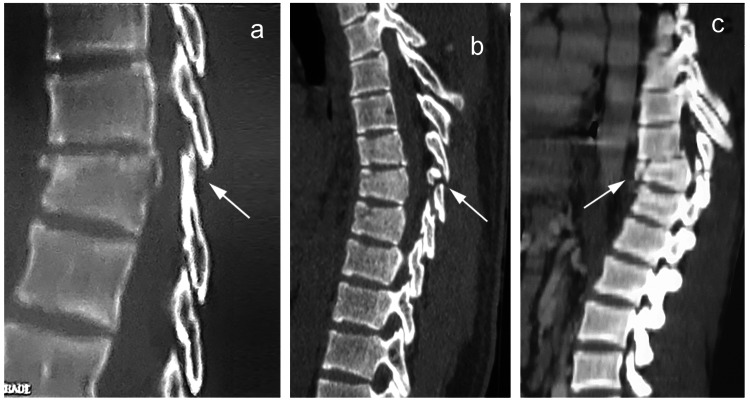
Indirect signs of posterior ligamentous complex injury on sagittal CT scans: (a) splaying of the spinous processes, (b) avulsion of the spinous process, and (c) translation of the vertebrae with associated fracture.
